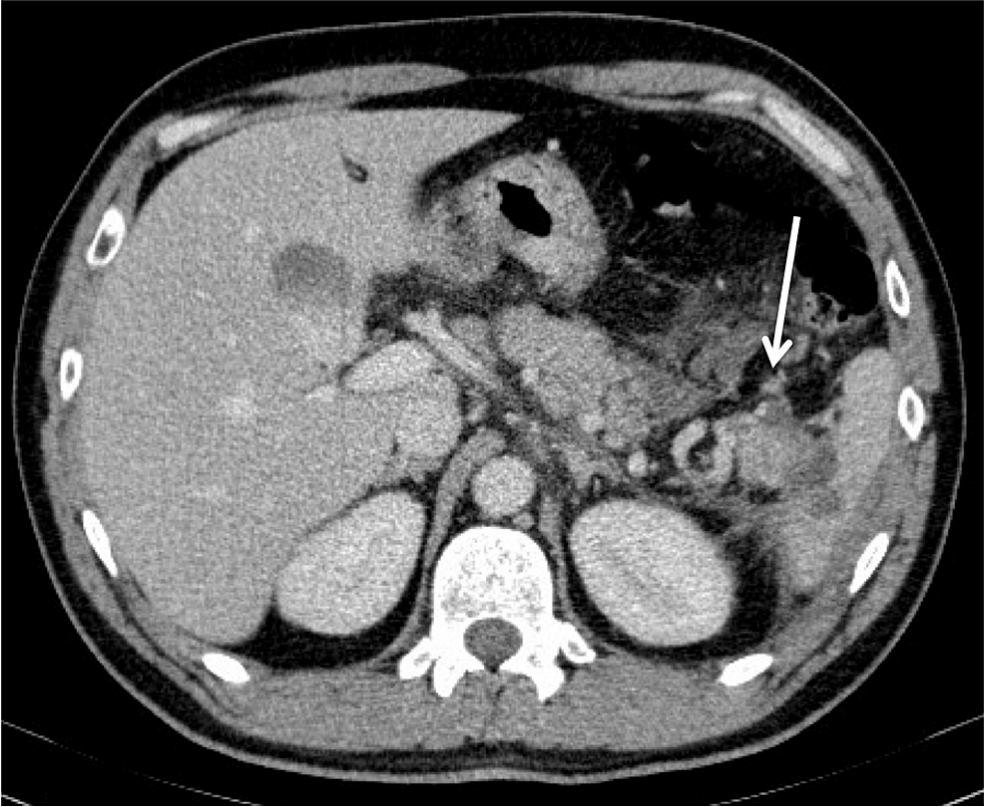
Contrast-enhanced CT scan (axial plane) 24 hours after admission showing splenic vein thrombosis.
